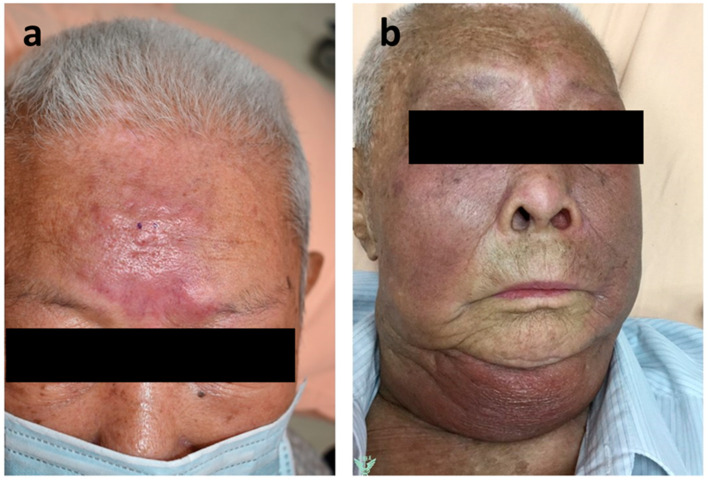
An 88-year-old Taiwanese male initially presented with ill-defined, erythematous patches over the forehead in November 2020. (a) Twenty months later, he developed confluent, erythematous patches from the head to the neck in September 2022. (b) Neck CT scans revealed diffuse, cutaneous, and subcutaneous infiltrations in the head and neck areas, being more prominent on the left side, with the imaging differential diagnoses of cellulitis, metastasis, among others. Subsequent neck sonography showed enlarged lymph nodes in the bilateral neck regions, up to 2.3 cm in size.
